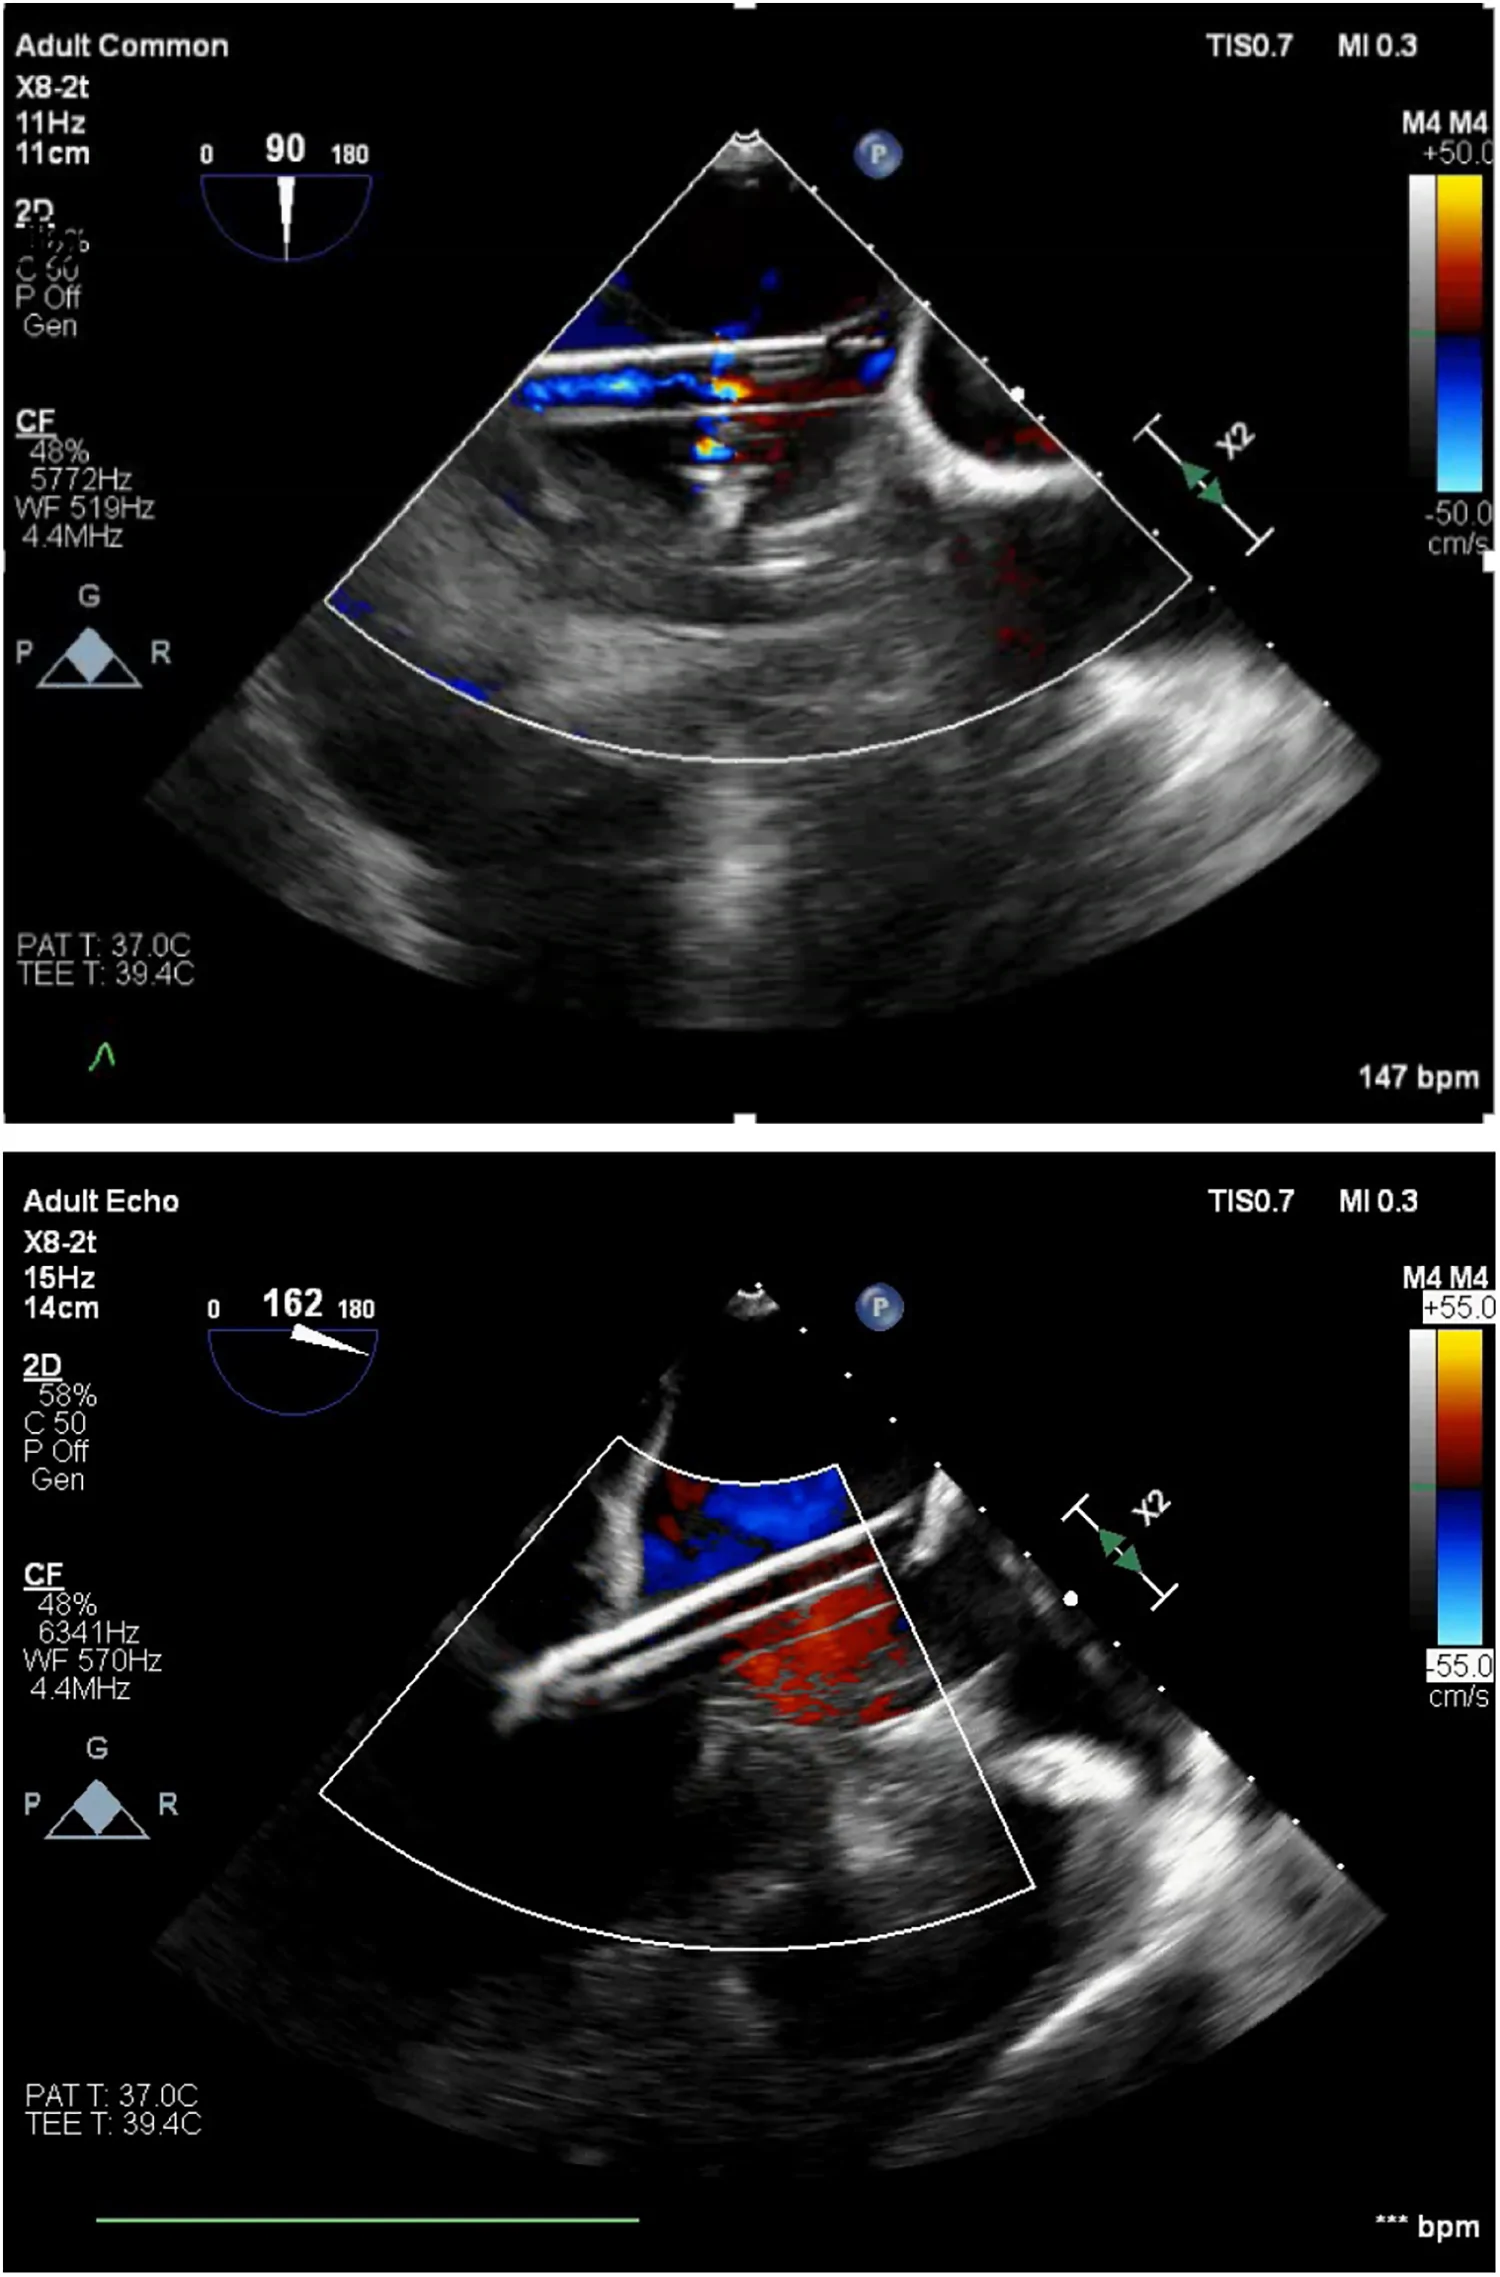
Transesophageal echocardiography after an LVAD insertion.
